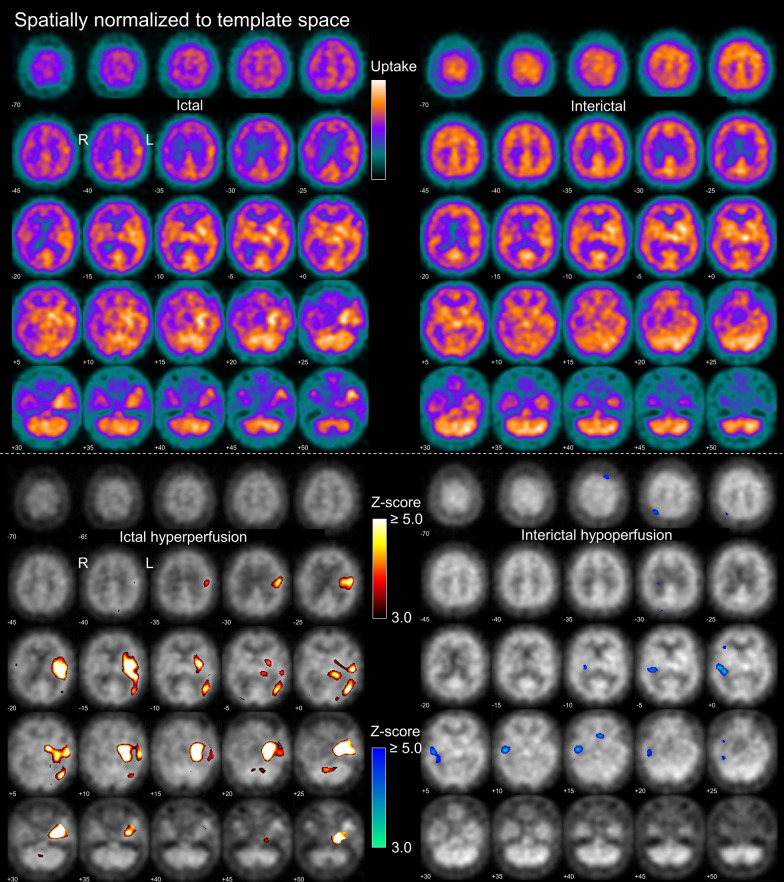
False lateralization by interictal SPECT. Ictal SPECT and interictal SPECT with.^99m^T-HMPAO in a 29 y old man with therapy refractory epilepsy and unclear lesion in the left temporal lobe (ictal SPECT: injection of 569 MBq 33 s after electrical seizure onset, post-injection seizure duration 40 s, start of SPECT acquisition 91 min after injection; interictal SPECT: injection of 534 MBq, start of SPECT acquisition 93 min after injection). In the ictal SPECT, the SOZ was lateralized to the *left* hemisphere with highest possible confidence (confidence score = 5 by all readers). In the interictal SPECT, the SOZ was lateralized to the *right* hemisphere with high confidence (confidence score = 4/5 by 1/2 readers). In the “full” setting, the SOZ was lateralized to the *left* hemisphere with low confidence (confidence score = 3/5 by 1/2 readers)
